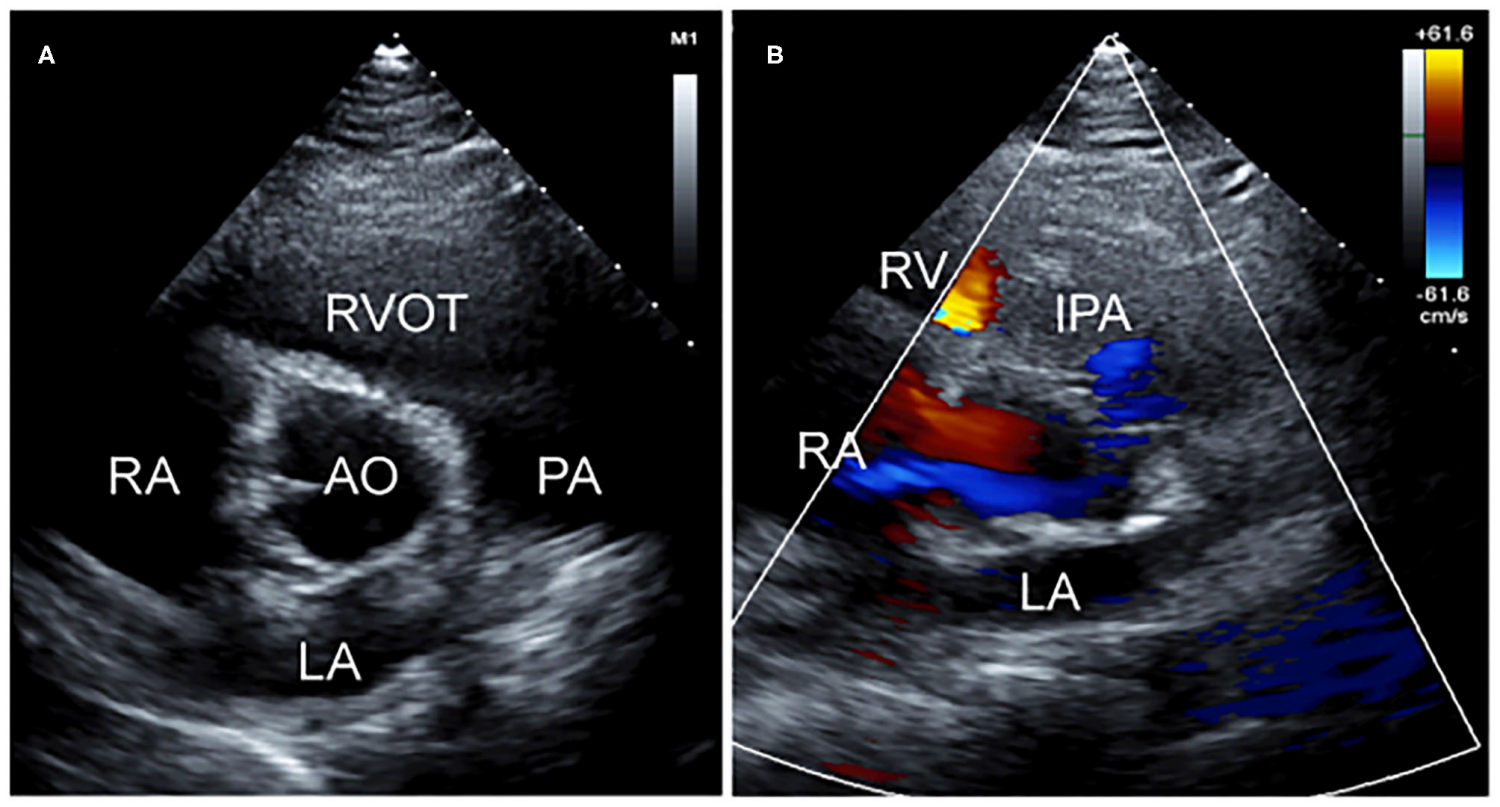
Post-operation two-dimensional transthoracic echocardiographic images. (A) Short-axis view at the aortic valve level showing the perforation of the left sinus of Valsalva repair and (B) non-standard short-axis view showing the cessation of abnormal blood flow on color Doppler.
